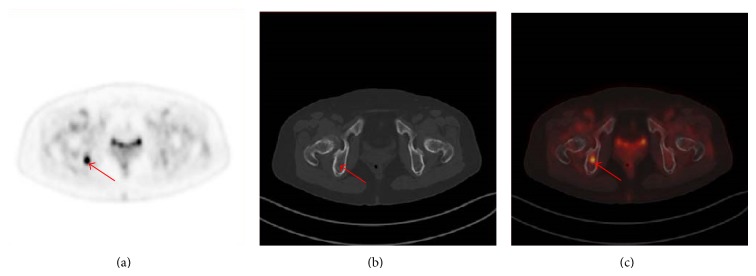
The PET image (c) demonstrates focal increased FDG uptake in right body of ischium (red arrow), which is considered as malignant. In the CT image (b), no abnormality is seen. The PET/CT (a) is considered as suspicious malignant because of the PET findings. Bone metastatic involvement of the right body of ischium was proved by follow-up.
